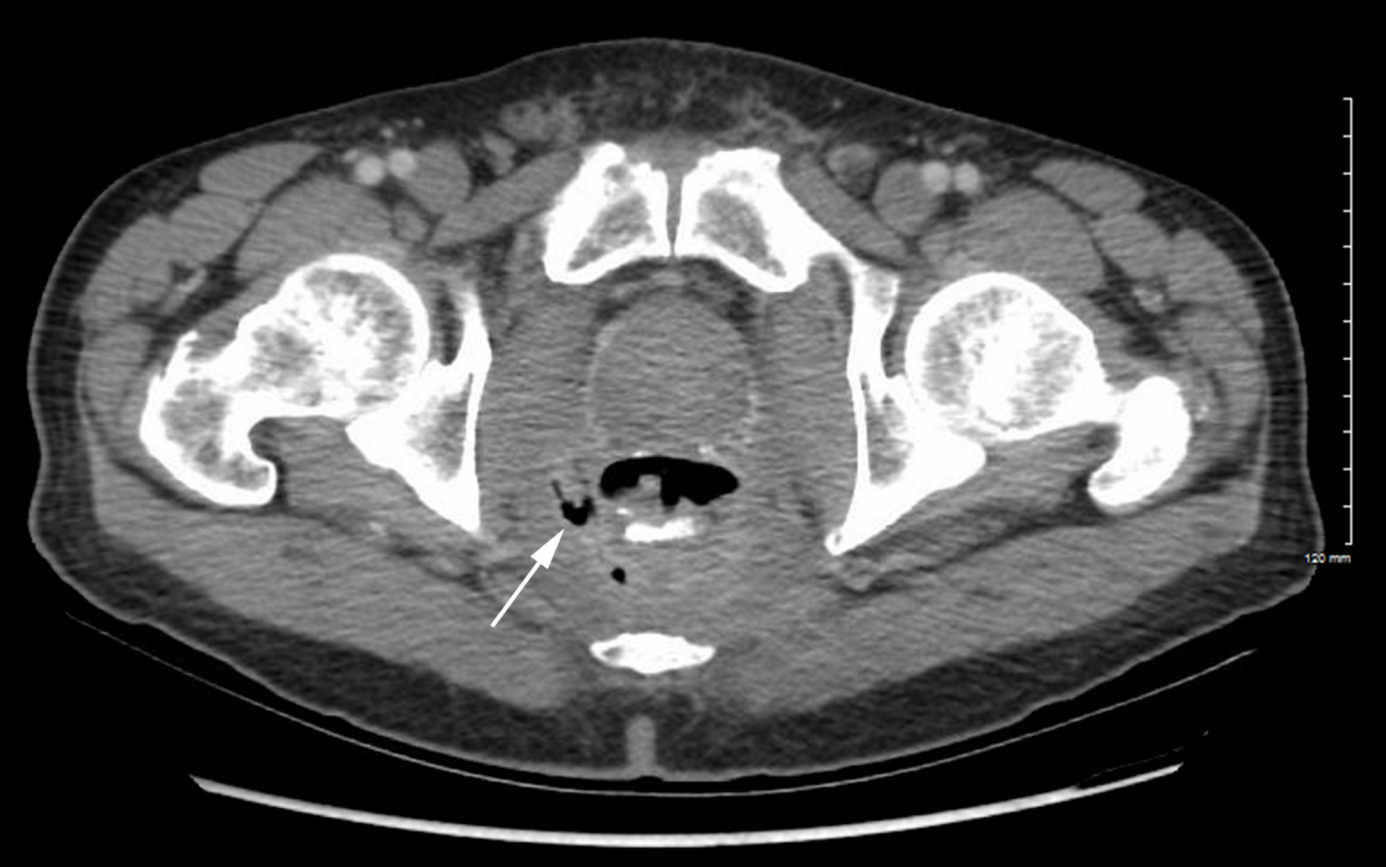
CT showing soft tissue and extraluminal air in the anastomosis
